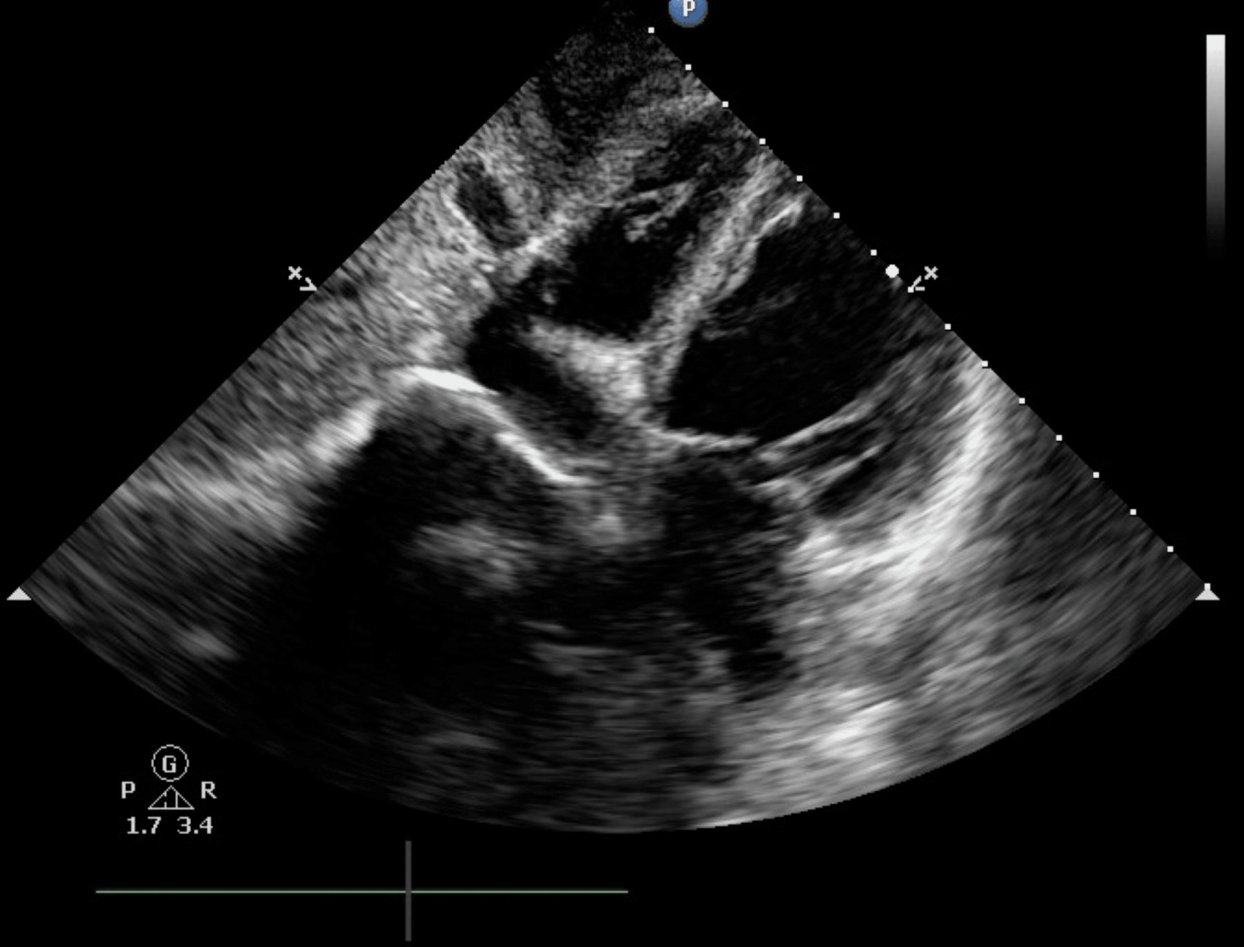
Subcostal TEE view revealing a prominent Eustachian valve within the right atrium TEE: transesophageal echocardiography
